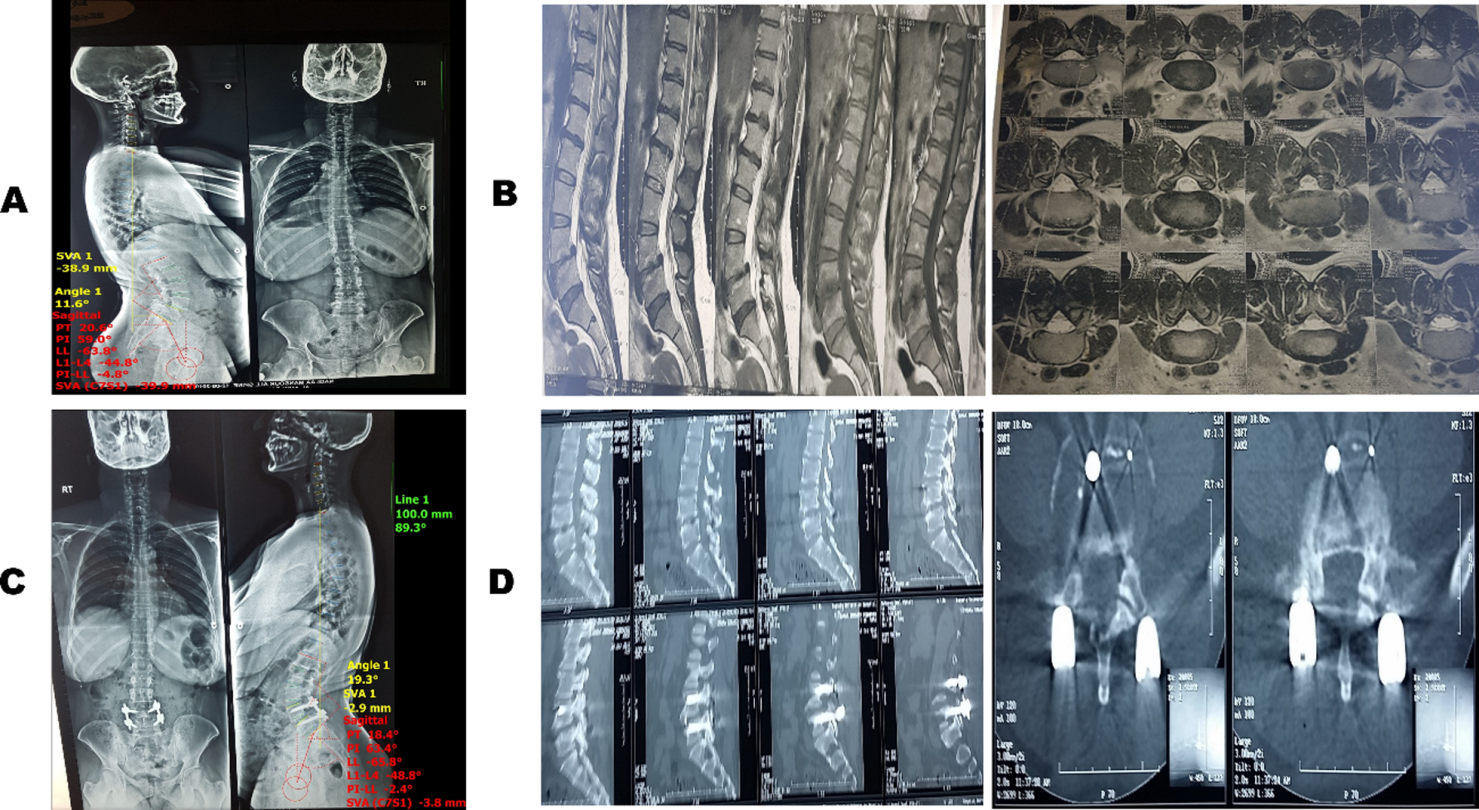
a Preoperative long-standing X-ray with spinopelvic parameters measurements, b preoperative MRI showing L4–5 isthmic spondylolisthesis, c postoperative long-standing X-ray with full correction and spinopelvic parameters measurements, and d postoperative follow-up CT at 1 year showing well union
